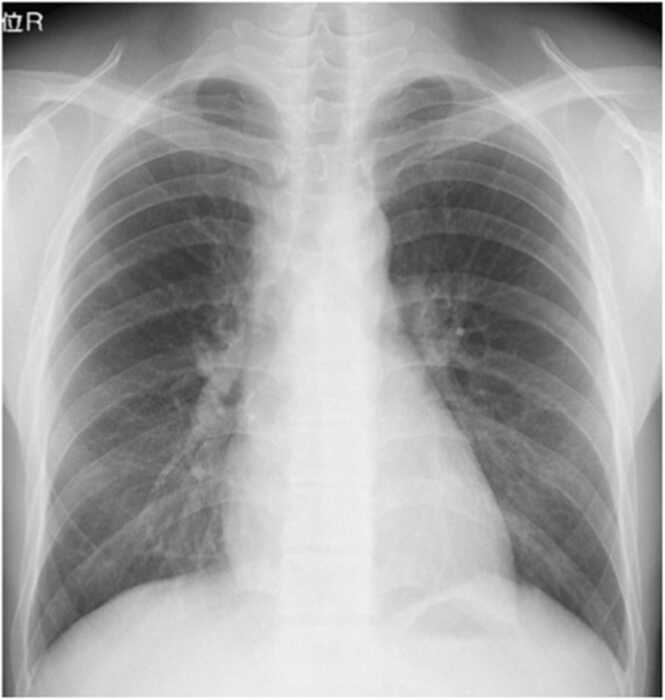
Chest X-ray after 1 month of steroid therapy showing improvement of pericarditis and mediastinal lymphadenitis.
